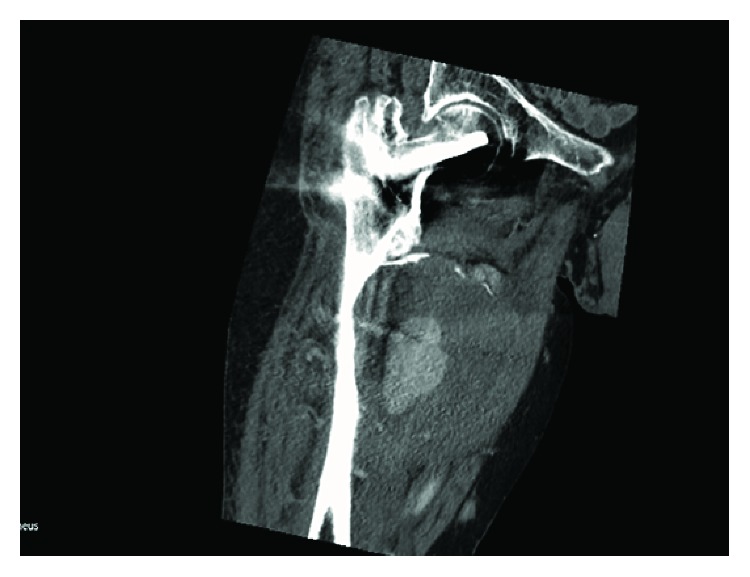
Contrast-enhanced computed tomography (CT) with IV contrast showed an active extravasation in the hematoma which concluded to the diagnosis of pseudoaneurysm. Severe scalloping of the medial femoral cortex was confirmed.
